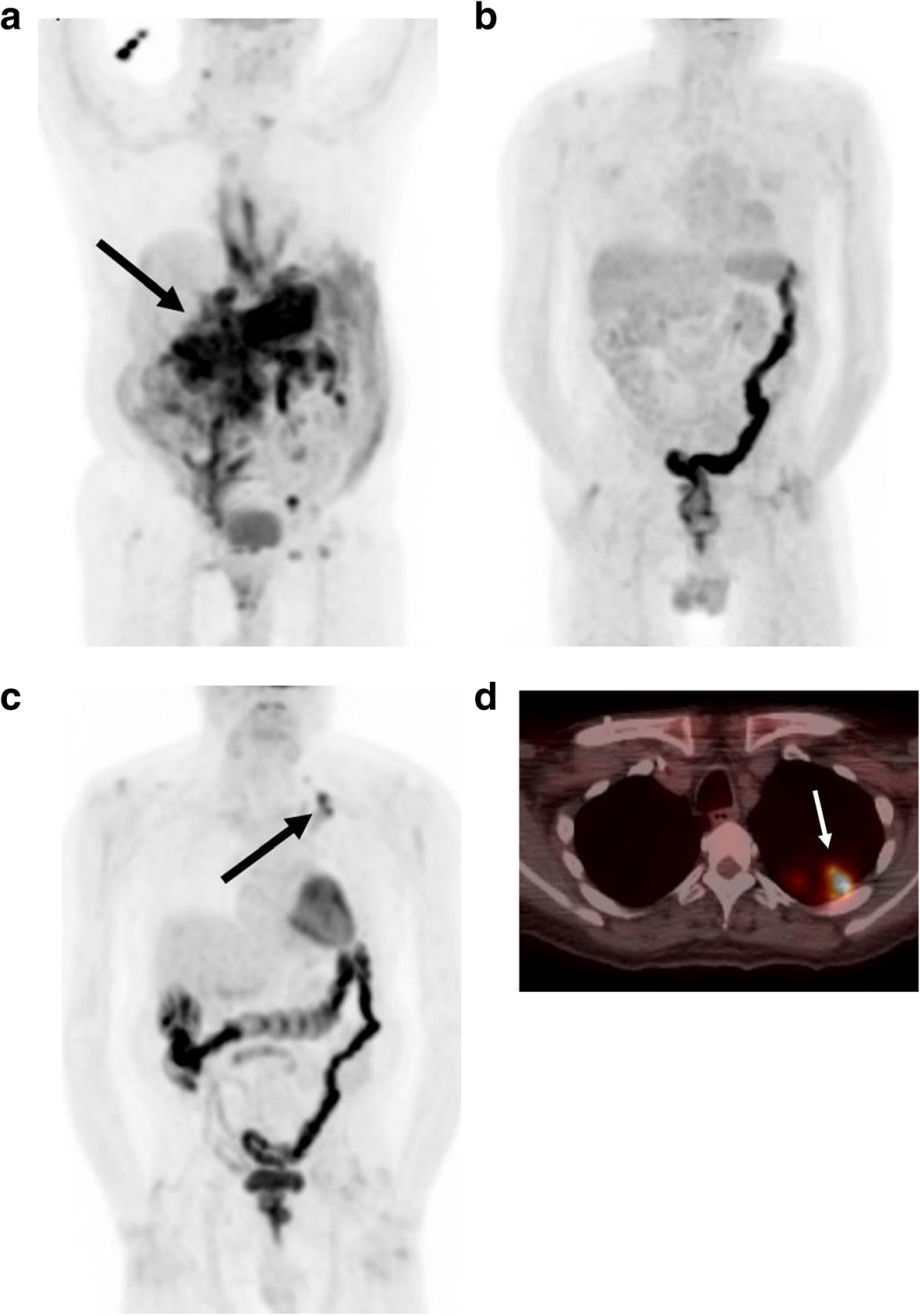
Pulmonary recurrence. An 83-year-old man initially presenting with GI bleeding and was found to have conglomerate gastric and intra-abdominal masses. a Initial staging whole body planar PET image demonstrates extensive abdominal disease involvement (arrow). He went on to complete 4 cycles of bendamustine and rituximab. b Follow-up whole body planar PET image demonstrates complete disease response. c A subsequent restaging whole body planar PET image demonstrates a new hypermetabolic focus within the left upper thorax (arrow). d Corresponding fused axial PET-CT demonstrates a pleural-based lesion (arrow). Percutaneous biopsy revealed Burkitt lymphoma
